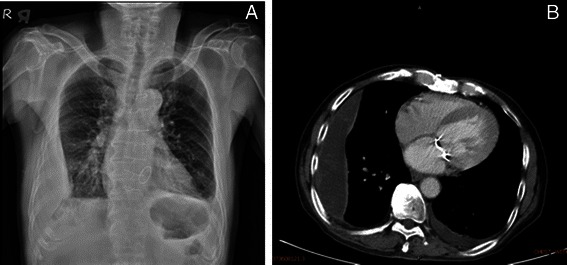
a Chest plain film generated at our emergency department showing the “fat sign” in the right lower thorax. b Chest CT showing a unilateral (right side) extrapleural hematoma
